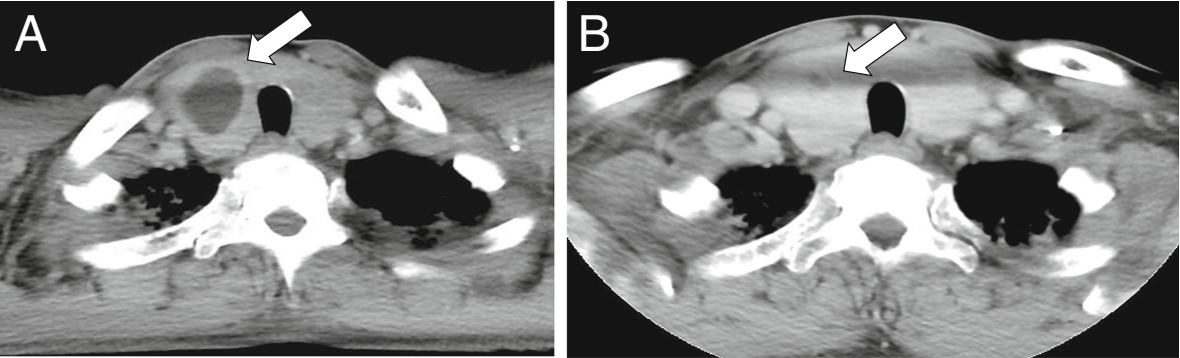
The thyroid gland findings on computed tomography (CT) scan. a. The systemic contact-enhanced CT scan on admission day 4 showing enlarged thyroid gland and low-density area in the right lobe (white arrow). b. The systemic contact-enhanced CT scan of the thyroid gland 5 months after admission showing the small remains of the thyroid abscess in the right lobe (white arrow)
